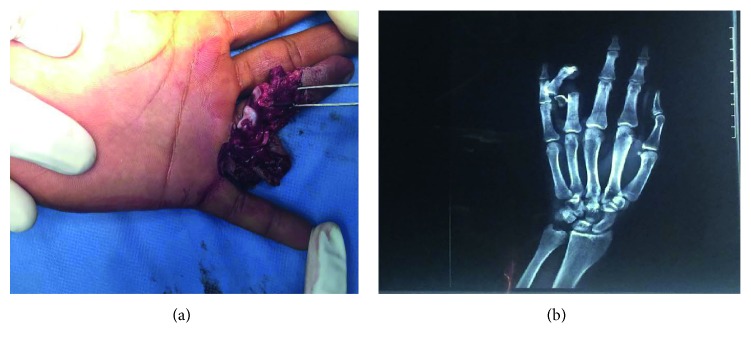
(a) Clinical aspect of the crush injury of the fourth finger. (b) X-rays showed a fracture dislocation of the proximal interphalangeal joint with third fragment.
